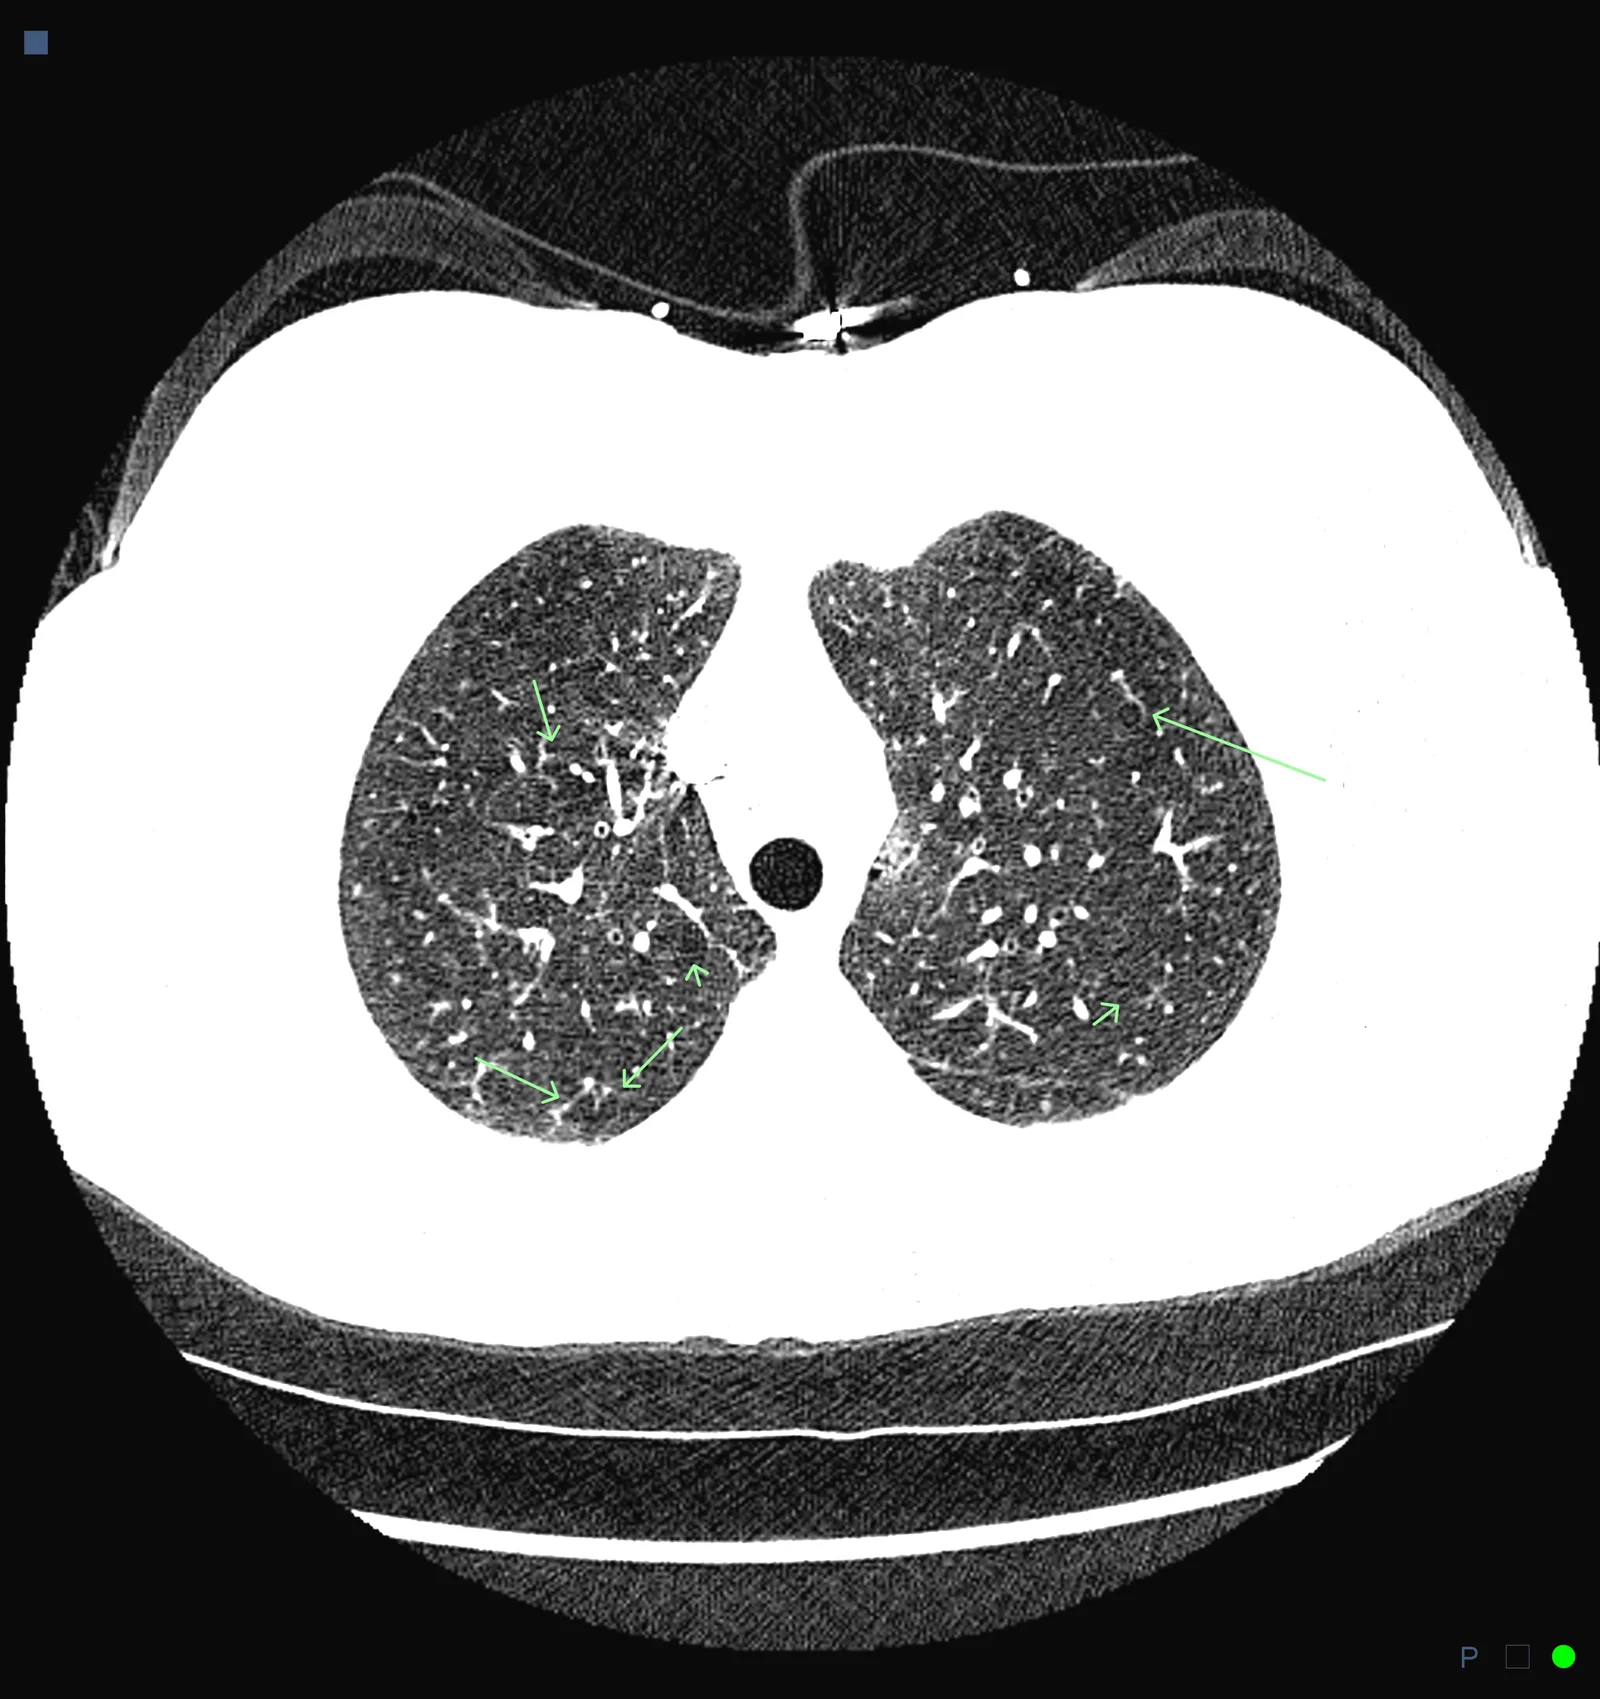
Axial CT pulmonary angiogram demonstrating bilateral centrilobular emphysema Mild centrilobular emphysema is present, affecting the proximal respiratory bronchioles, characterized by round, lucent foci (arrows) involving the central pulmonary lobular unit with upper-lobe (apical) predominance. Mild centrilobular emphysema involves 0.5%-5% of the lung zone.
